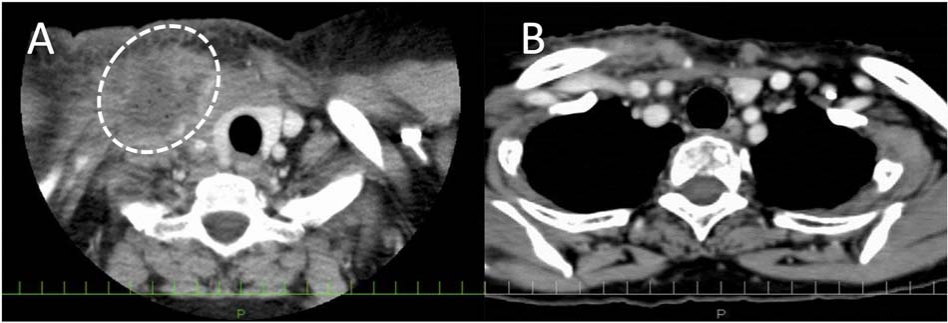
Enhanced CT before and after OK432 injection. (A) Before OK432 injection. The lymph (dashed circle) is retained in the right supraclavicular fossa. (B) After injection. The lymphatic leakage disappeared after OK432 sclerotherapy.
